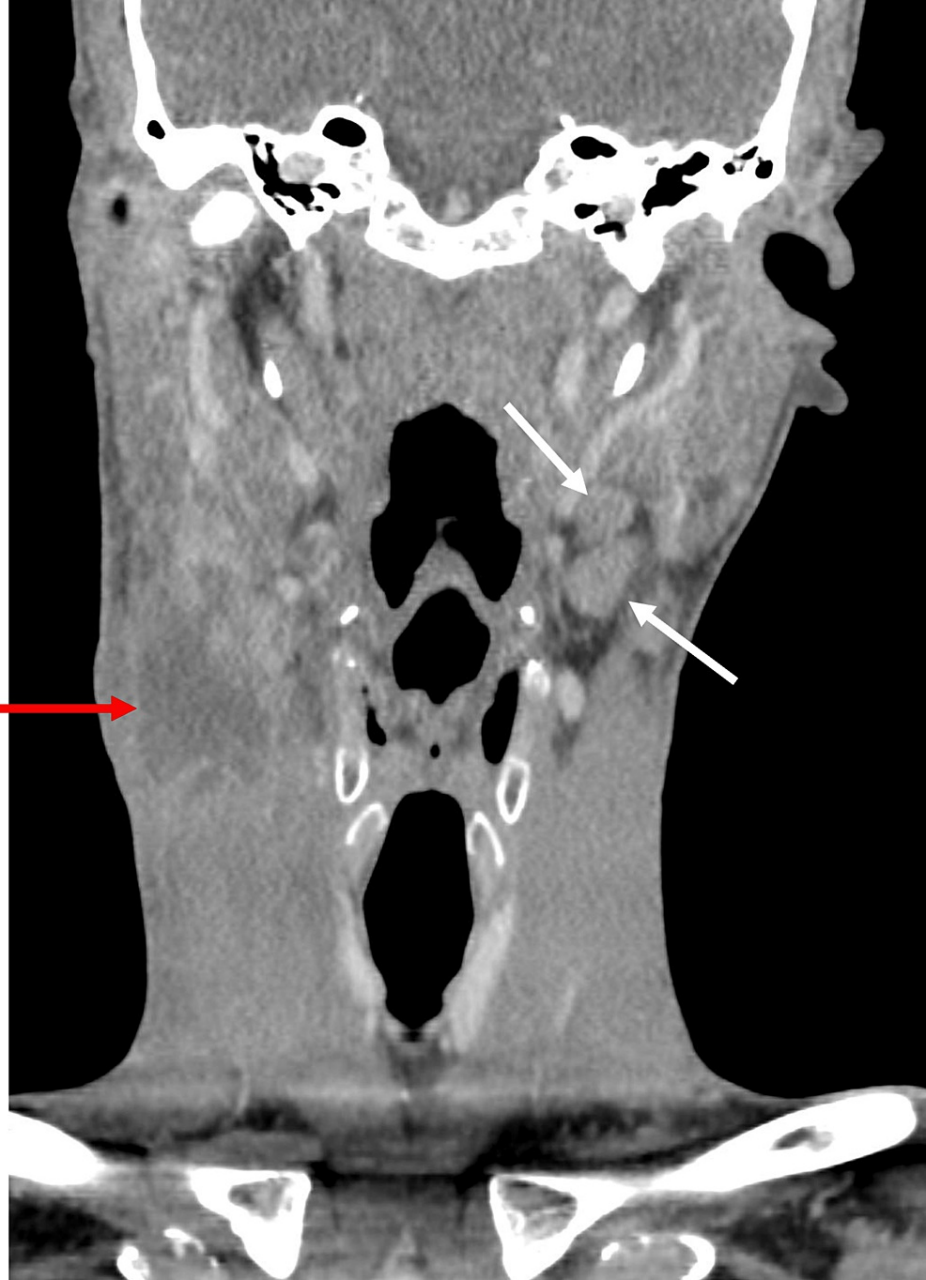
Coronal reformat CT of the neck (soft tissue) CT of the neck (soft tissue) with contrast coronal reformat demonstrated a large conglomerate right level 2A/3 lymph node with internal areas of non-enhancement (red arrow). There are additional enlarged left cervical lymph nodes (white arrows).
